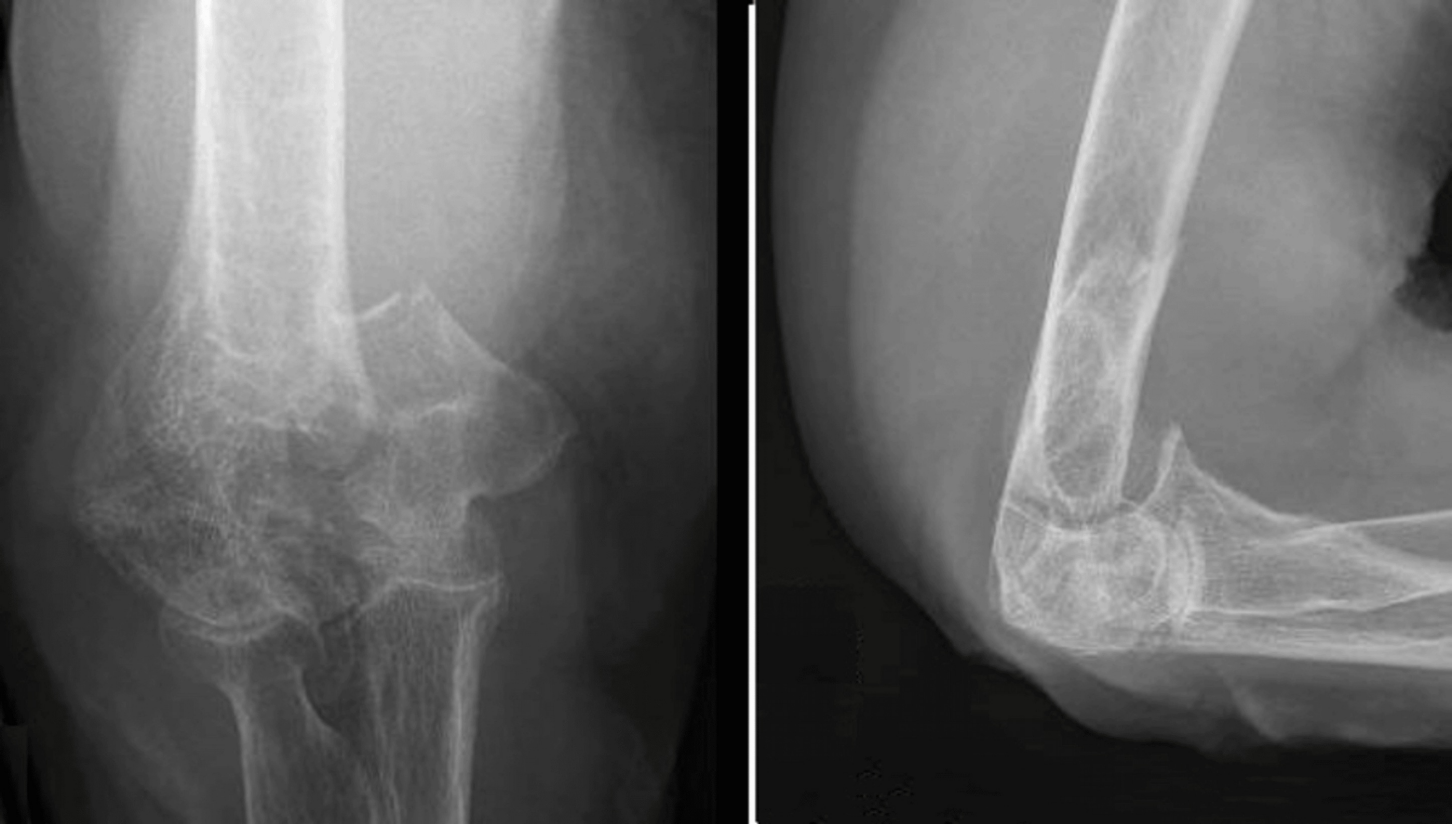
Preoperative radiograph of a patient's elbow who underwent DHH later. DHH: distal humerus hemiarthroplasty
